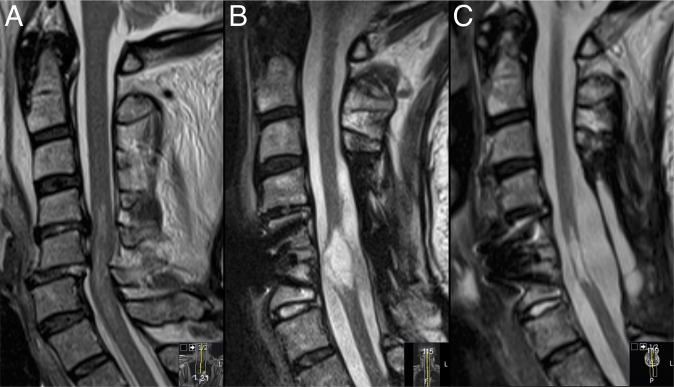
Time points in a  case of PSCT. Sagittal T2 magnetic resonance images immediately after trauma (A), tethering and syringomyelia formation 3 years after trauma (B), and following surgical untethering (C).
